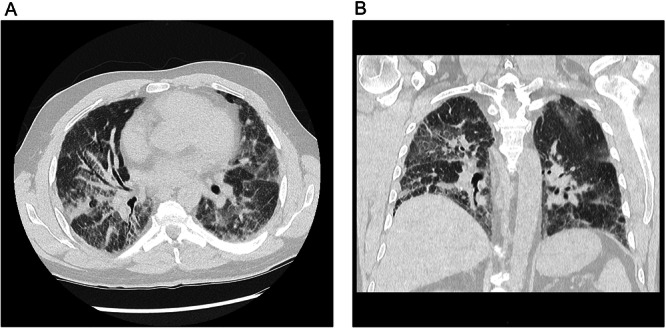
Chest computed tomography (CT) of Patient 4 (2024, before bilateral lung transplantation) in axial (A, mid‐to‐basal) and coronal (B) planes, lung window. Findings: Ground‐glass–like infiltrates, micronodules, diffuse pulmonary fibrosis, and cardiomegaly.
